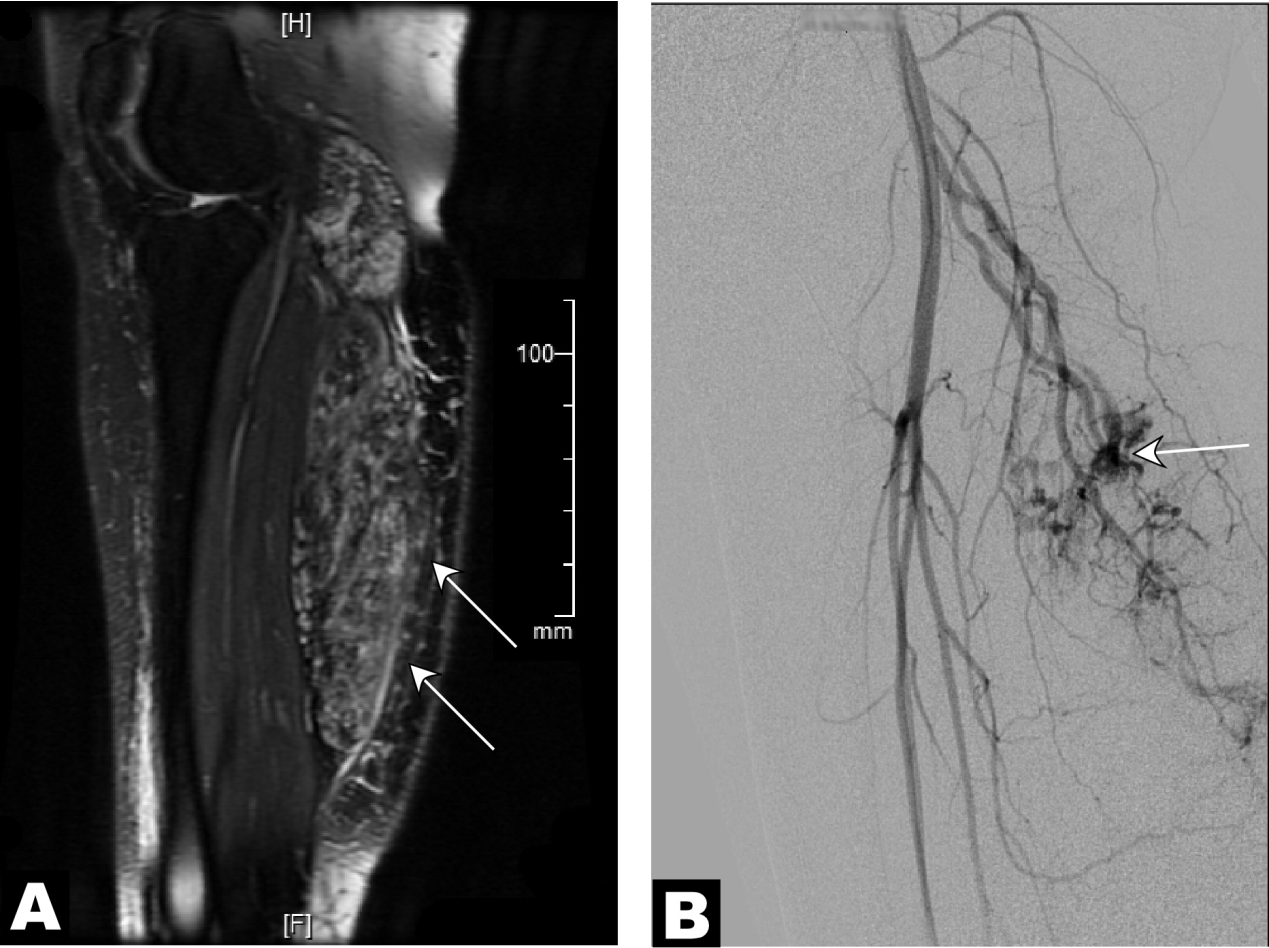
MR and angiographic studies suggestive of multiple arteriovenous shunts supplying a venous malformation. A 26-year-old female with a painful vascular malformation of the right lower leg with a clinically obvious venous component was evaluated for arteriovenous shunting. (A) Parasagittal T2-weighted MR image with intravenous gadolinium contrast demonstrated an infiltrative vascular malformation in the right gastrocnemius (*arrows*). (B) Digital subtraction angiogram of the right leg in a lateral projection during the arterial phase revealed arteriovenous shunting (*arrow*) within the vascular malformation, supplied by sural branches of the right popliteal artery.
